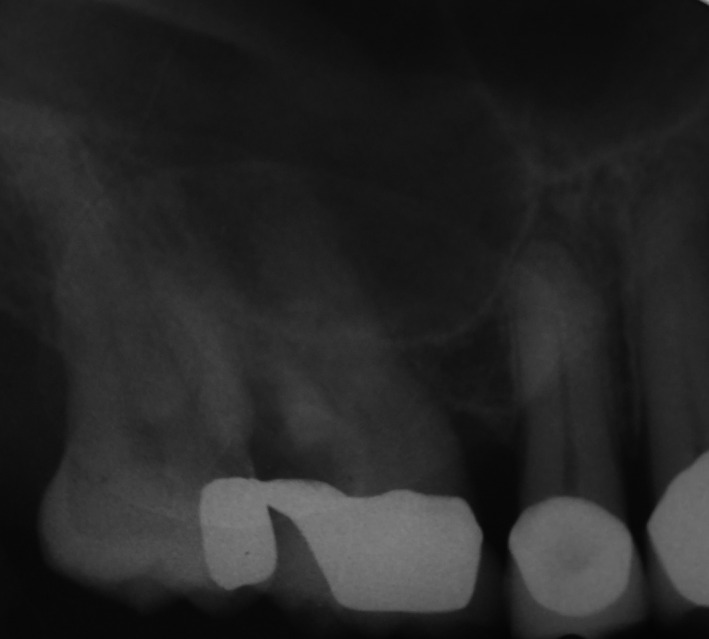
Preoperative radiograph of the unrestorable maxillary right first molar tooth
